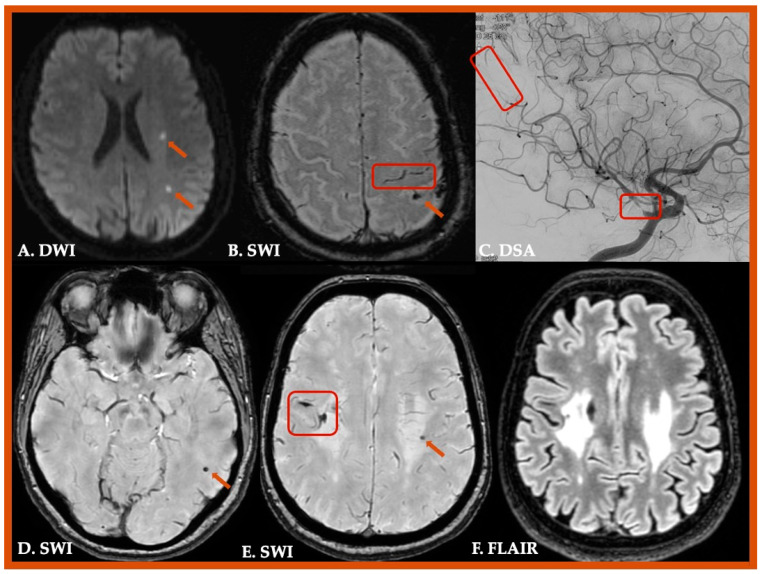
Radiological mimics of Cerebral Amyloid Angiopathy. A 55-year-old male patient with multiple cerebral microinfarcts (Panel (A)), left parietal cortical superficial siderosis and lobar microbleeds (Panel (B)) attributed to primary angiitis of the Central Nervous System. Digital subtraction angiography revealed multiple intracranial stenoses in both proximal and distal arteries (Panel (C)). A 57-year-old female patient with multiple lobar and deep hemorrhagic lesions (Panels (D,E)) associated with confluent, bilateral, symmetric white matter hyperintensities on FLAIR sequences, with relative sparing of subcortical U-fibers (Panel (F)). Genome wide sequencing revealed the pathogenic mutation NM_001845.6:c.2245G>A, p.(Gly749Ser), leading to the diagnosis of COL4A1-related disorder.
